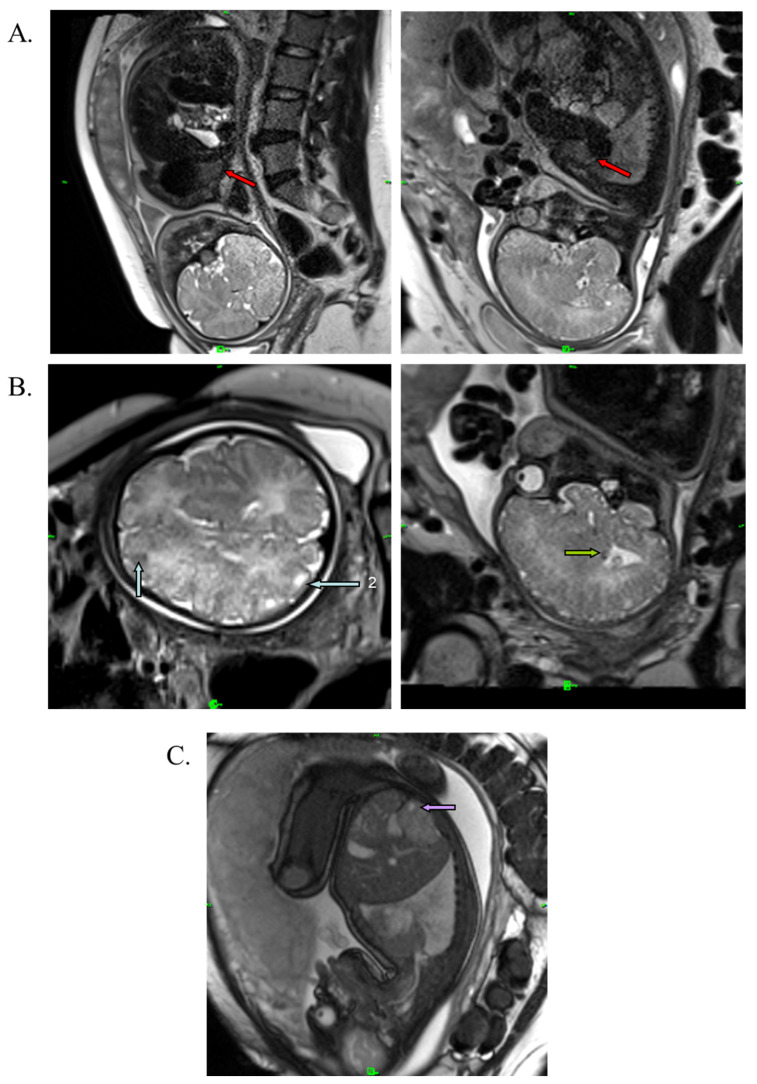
T2-weighted MRI image of the fetus at the 38th week of gestation. (A) Red arrows show cardiac rhabdomyoma in reduced size (20 × 20 × 33 mm). (B) Blue arrows show cortical tuber in the gyral core (2), and green arrows represent subependymal nodule in the brain. (C) Purple arrow shows the renal cyst.
